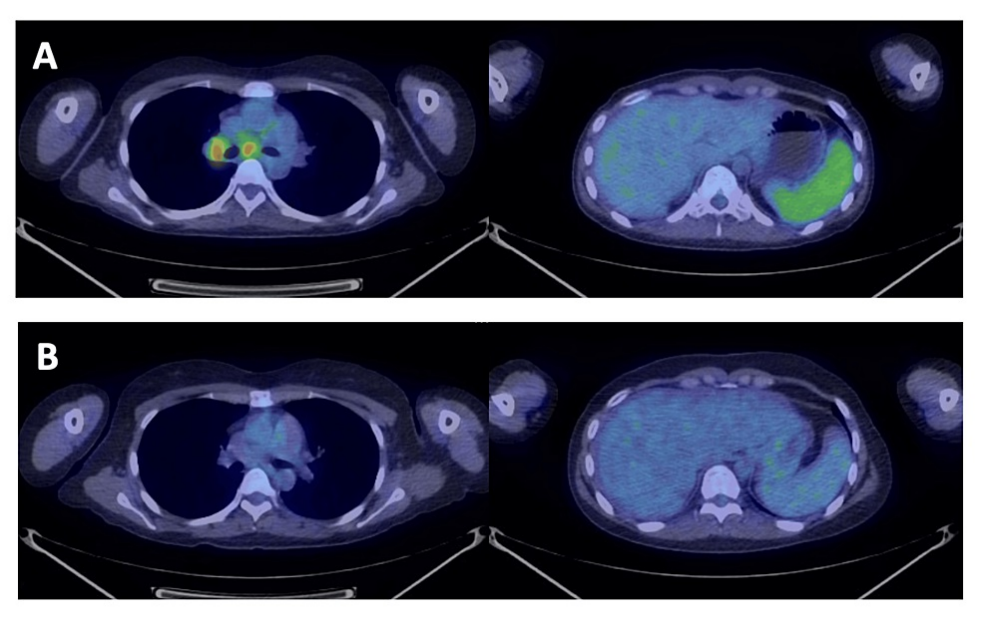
18F-FDG PET/CT. (A) A PET/CT scan revealed FDG uptake in the enlarged mediastinal and hilar lymph nodes and spleen. The patient relapsed six months after autologous stem cell transplant. (B) A PET/CT scan after 16 cycles of brentuximab vedotin demonstrated a complete metabolic response. 18F-FDG: fluorine-18 fluorodeoxyglucose, PET: positron emission tomography.
